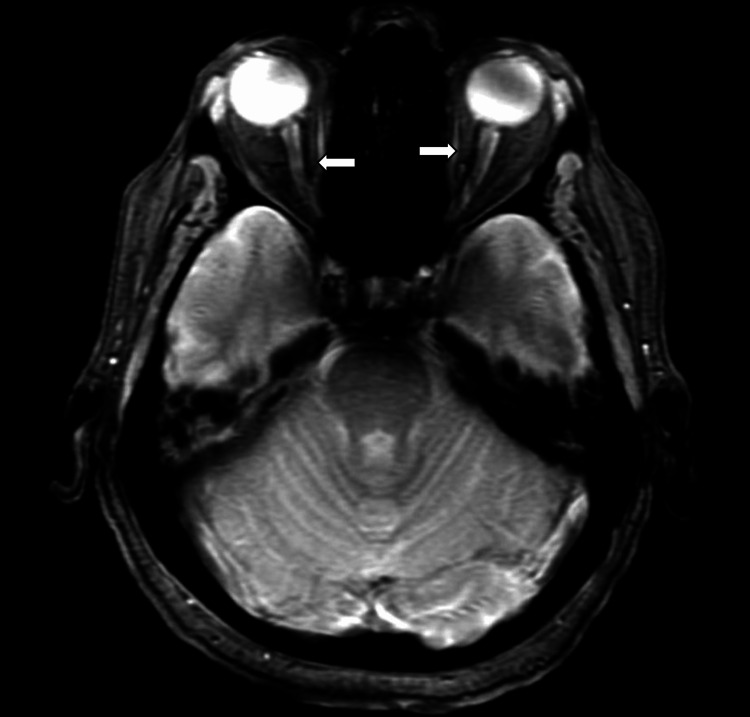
Orbit MRI showing increased CSF MRI: magnetic resonance imaging; CSF: cerebrospinal fluid
